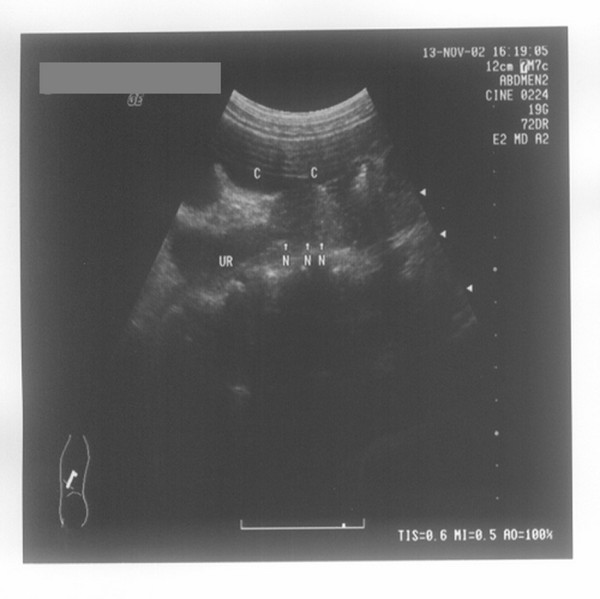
Ureter stricture. Seen under transabdominal ultrasound after normal saline retention enema, stricture (indicted by N) of the left ureter (indicted by UR) is visible behind the splenic flexure of the colon and upper descending colon (indicted by C).
